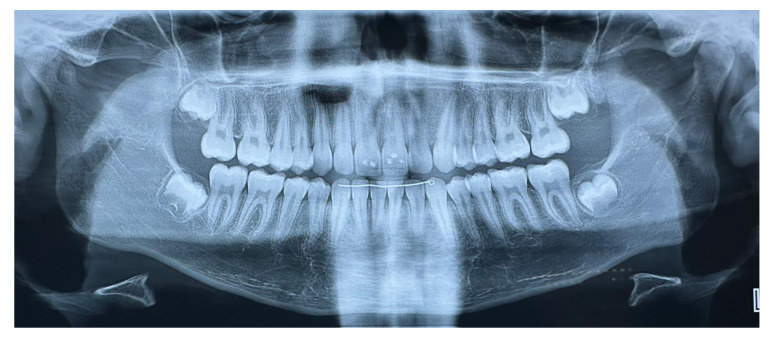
Orthopantomography at T1.
